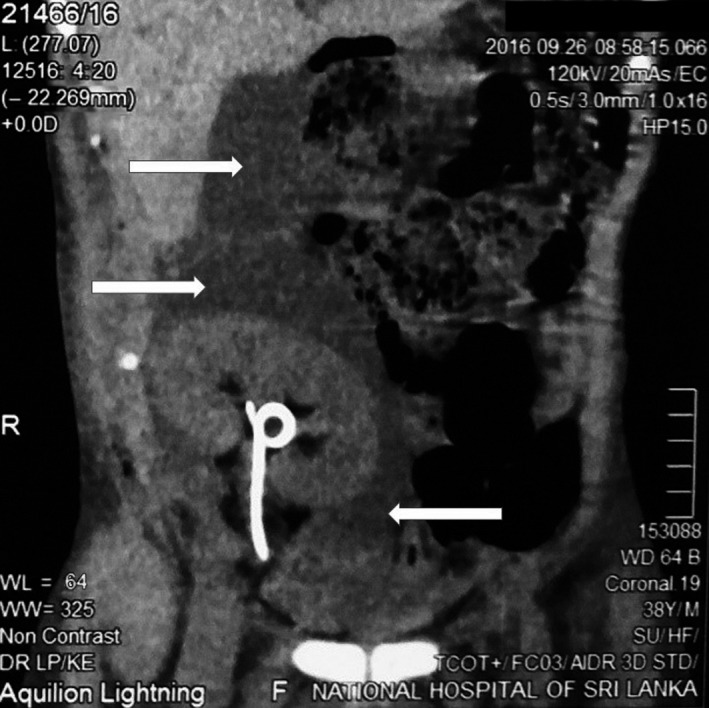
Noncontrast Computed tomography scan showing homogenous fluid collection (white arrows) posterior to the transplanted kidney which extends superiorly up to the right subhepatic area and inferiorly into the pelvis, displacing the bladder to the left
